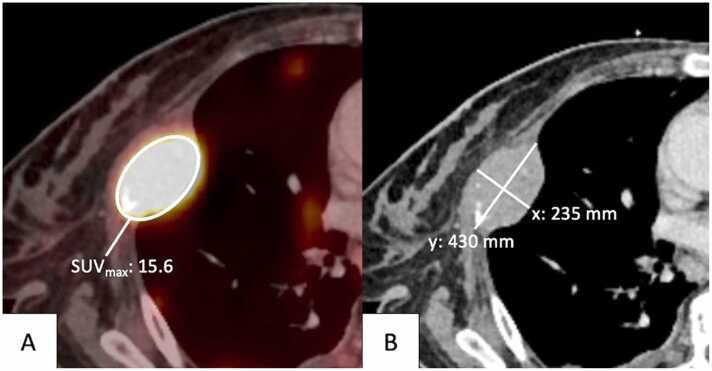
[^18^F]FDG-PET/CT examination of a fifty-one-year-old patient with DLBCL and pleural lymphoma manifestation and infiltration of adjacent rib. Example of image analysis with (A) measurement the SUV_max_ in the tumor area with the highest metabolic activity using a volume of interest (VOI), and (B) measurement of maximal (y) and minimal (x) diameters in mm.
